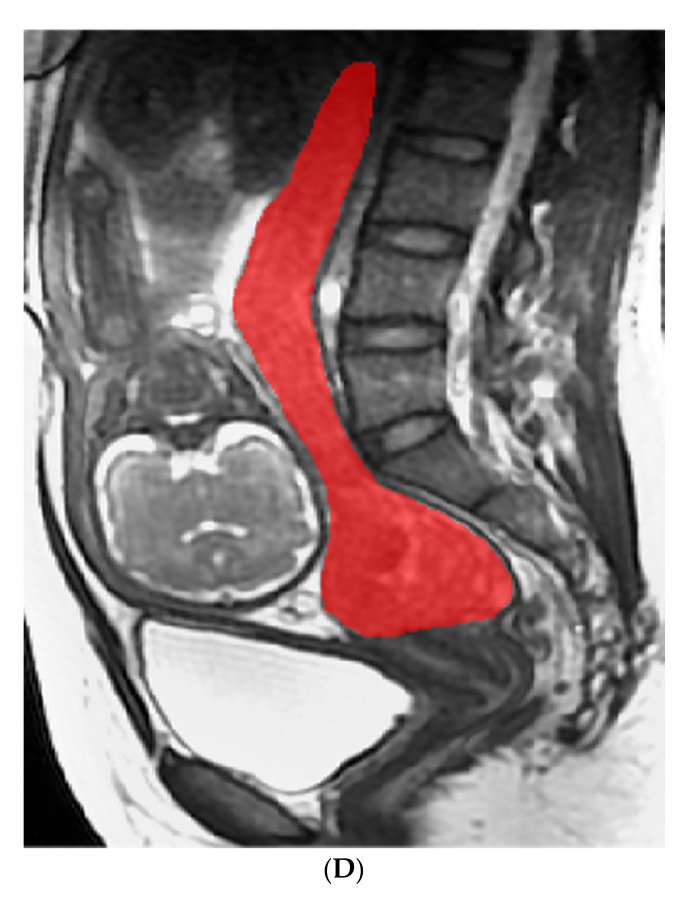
(A,B): A 36-year-old patient with placenta increta, receiving manual stripping of the placenta along with the amount of IBL of up to 5000 mL. The red region shows the VOI of delineation including the placenta and uterus; Weeks of gestation at time of MRI examination: 35.4/10 points; Placenta previa: Complete/42 points; Rad score: 0.64/58 points; Total score: 110 points; The risk of manual stripping placenta: 0.9; Number of CS: 1/48 points; Placenta previa: Complete/88 points; Rad score: 3.2/100 points; Total score: 236 points; The risk of the amount of IBL more than 1000 mL: >0.9; (C,D): A 23-year-old patient with placenta accreta, who underwent active separation of the placenta along with the amount of IBL of 350 mL. The red region shows the VOI of delineation including the placenta and uterus; Weeks of gestation at time of MRI examination: 30.9/20 points; Placenta previa: Partial/28 points; Rad score: −2.4/0 points; Total score: 48 points; The risk of manual stripping placenta: 0.2; Number of CS: 0/0 points; Placenta previa: Partial/60 points; Rad score: −0.76/10 points; Total score: 70 points; The risk of the amount of IBL more than 1000 mL: 0.15.
